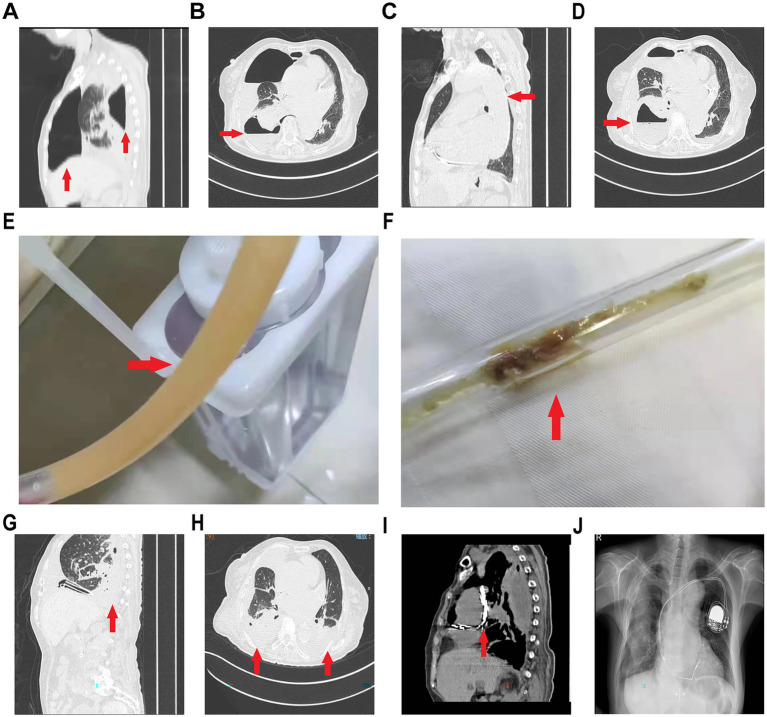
Serial thoracic imaging of the patient. (A,B) Day 1 post-procedure CT images (sagittal and axial views) demonstrate right-sided hydropneumothorax and pulmonary atelectasis. (C,D) Day 5 CT images (sagittal and axial views) show worsening right pleural effusion and consolidation. (E,F) Photographs of the chest tube drainage, which appeared turbid with foul odor and contained particulate debris. (G,H) Day 13 CT (sagittal and axial views) shows significant resolution of pleural effusion and pulmonary consolidation. (I) Day 52 contrast-enhanced upper gastrointestinal CT (axial view) confirms the closure of the esophageal-pleural fistula, with no evidence of contrast extravasation into the thoracic cavity. (J) Chest X-ray at 3 months shows complete pulmonary re-expansion. (Red arrow highlights the relevant pathological findings).
